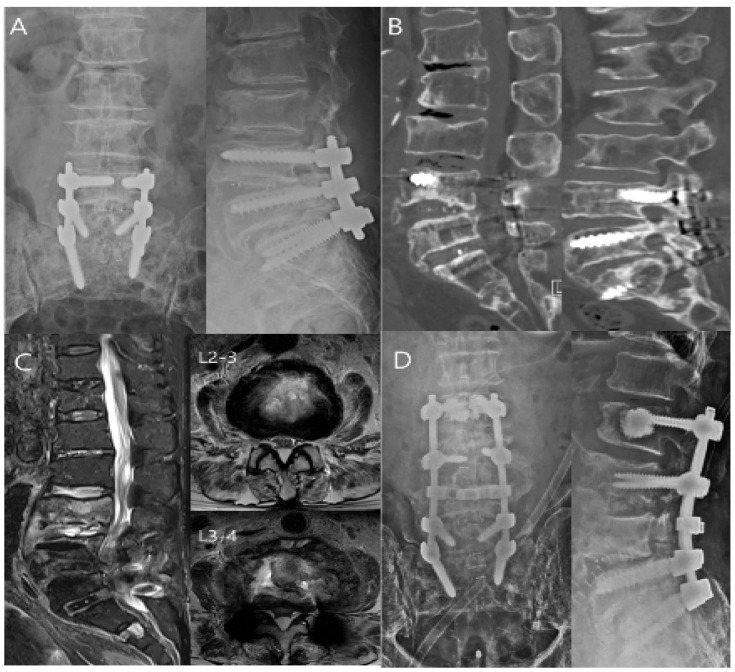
A 70-year-old woman with L4–L5–S1 spinal stenosis underwent partial laminectomy, posterior lumbar interbody fusion, and instrumentation. After four months, she returned with sudden severe back pain. The surgical site exhibited swelling and warmth, resulting in a suspected and confirmed late-postoperative infection via X-ray and CT scan (A,B). No evidence of implant loosening was observed. An initial incision and drainage surgery provided temporary relief, but signs of infection persisted, and the MRI revealed spondylodiscitis and worsening kyphotic deformity (C). She ultimately underwent revision surgery, including screw removal and extended fusion to L2 (D). The patient consented to publish her clinical images.
